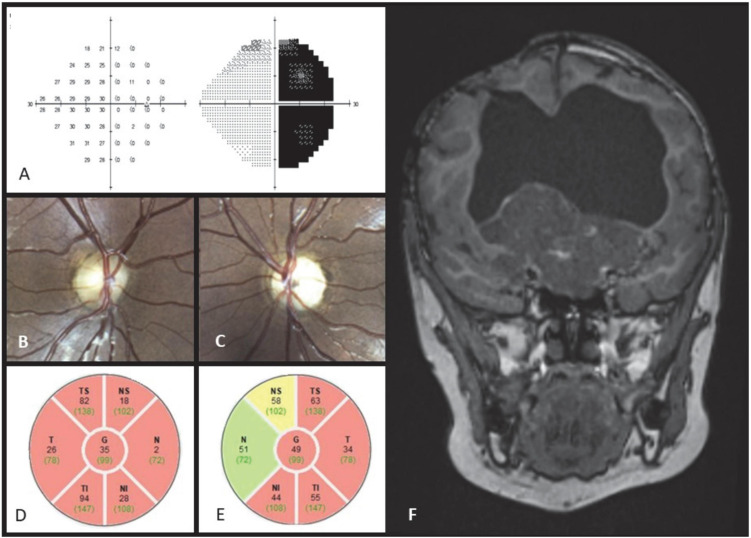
Case 6. Automated perimetry demonstrating a temporal hemianopia in the right eye (A). Fundus color photograph shows bow tie atrophy of the right optic disc (B) and diffuse atrophy of the left optic disc (C). OCT scan showing bilateral diffuse RNFL thinning, which is more profound in the nasal and temporal quadrants of the right optic disc (D,E). MRI T1 post contrast coronal scan showing massive suprasellar enhancing lesion and severe hydrocephalus (F).
